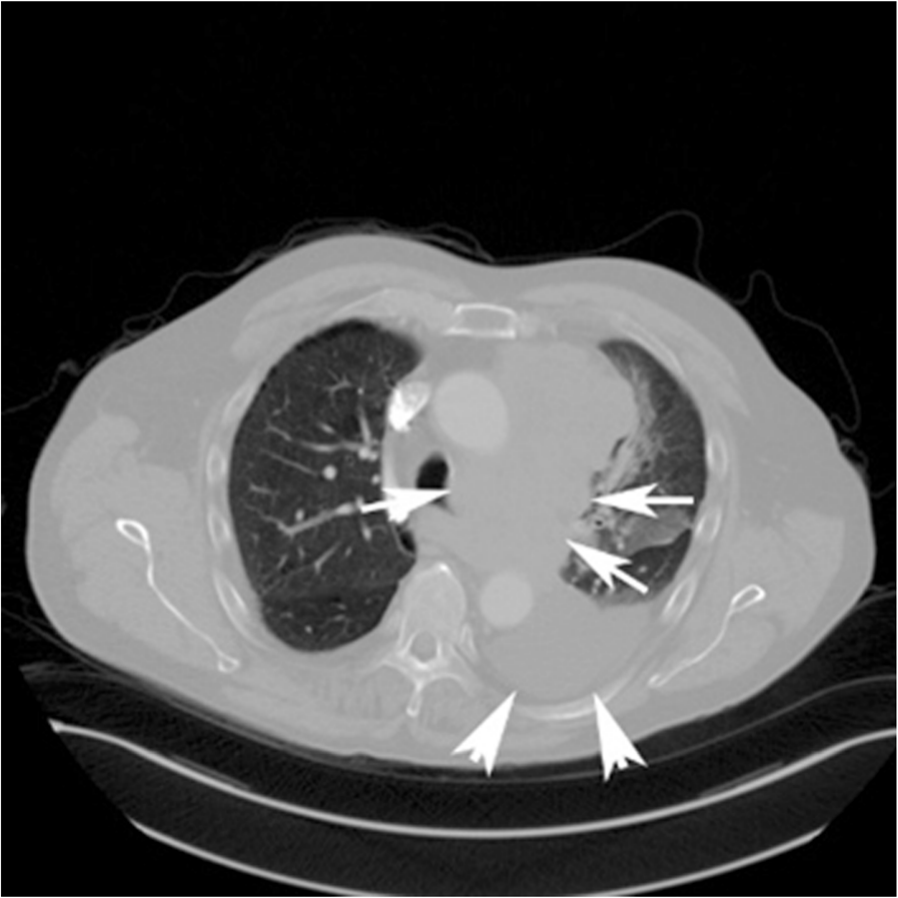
CT scan of the chest. CT of the thorax (horizontal section) showing a large mass measuring 4.6 × 12.0 × 8.1 cm, encasing the left pulmonary artery and segmental branches (arrows).The mass also abutts the left mainstem bronchus with partial encasement. Moderate-sized left pleural effusion (arrow heads).
